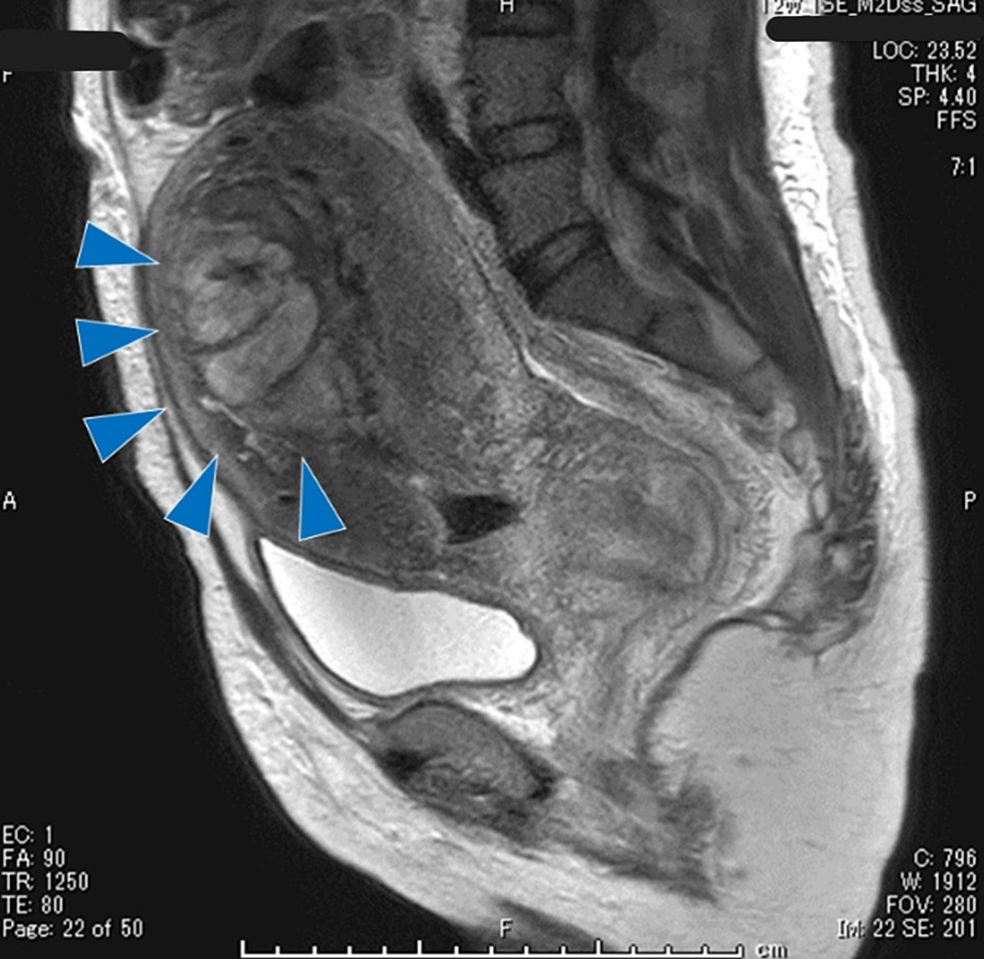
MRI for the diagnosis of placenta accreta MRI indicating a heterogeneous mass protruding into the endometrial cavity with high- and low-signal intensity on the T2-weighted image. The mass, 55×45×75 mm in size (arrows), was located from the bottom to the corpus of the uterine cavity. MRI: magnetic resonance imaging
